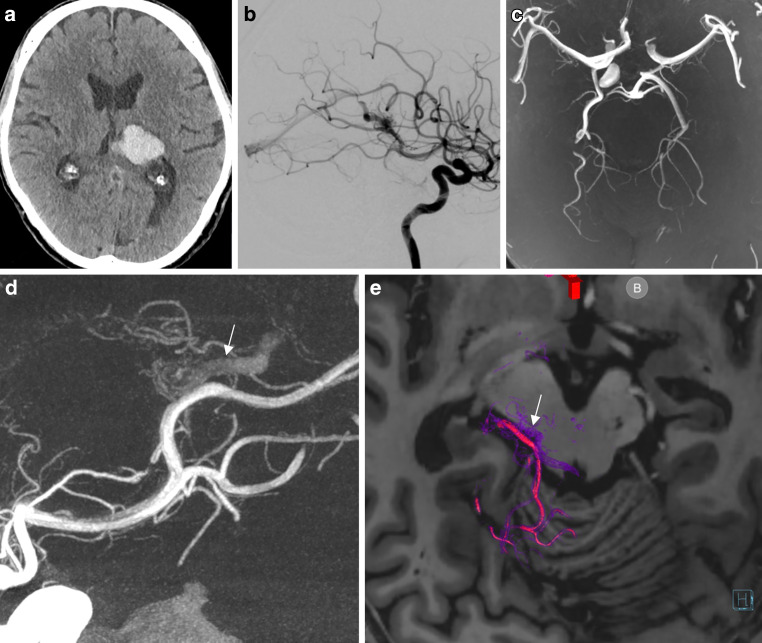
Small arteriovenous malformation (AVM) on the superficial surface of the mesencephalon after radiosurgery. a The baseline computed tomography (CT) scan demonstrating a left thalamic hemorrhage. b Digital subtraction angiography (DSA) showing the nidus and en-passant feeders from the left P3 segment and deep venous drainage. c Three years after radiosurgery, 7‑Tesla magnetic resonance imaging (MRI) does not show evidence of any abnormal vessels on the arterial time-of-flight sequence. d DSA demonstrates a residual arteriovenous shunt with early arterial filling of the posterior segment of the basal vein of Rosenthal (*arrow*). e Fusion of the follow-up 7‑Tesla and three-dimensional rotational angiography (3DRA) volumes clearly demonstrates the small nidus localized on the superficial surface of the posterolateral mesencephalon with a single draining vein corresponding to the posterior segment of the basal vein of Rosenthal (*purple*). Supplementary video demonstrates the fusion with an embedded 3D model of the AVM
